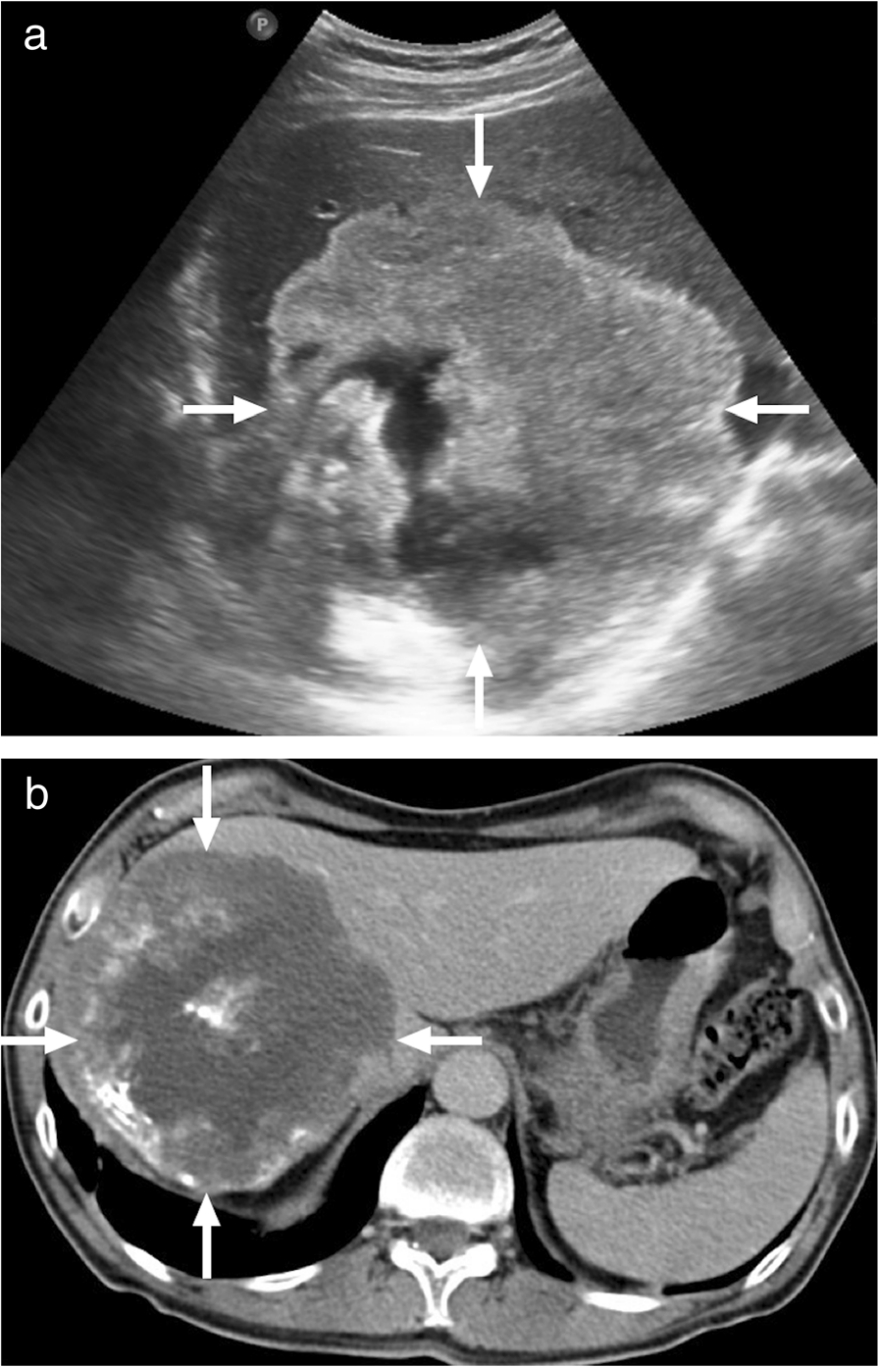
A 65-year-old male recently presented with right upper quadrant pain and abnormal liver function tests. a Abdominal ultrasound demonstrates lobulated, centrally cystic mass (arrows) within the right liver lobe. The mass was mostly hyperechoic in echotexture. b Axial plane postcontrast CT demonstrated a large, hypoattenuating, centrally cystic/necrotic liver mass (arrows) with scattered calcifications. Percutaneous biopsy was performed to exclude cholangiocellular cancer. Final diagnosis was alveolar hydatid cyst. The patient underwent right hepatectomy
